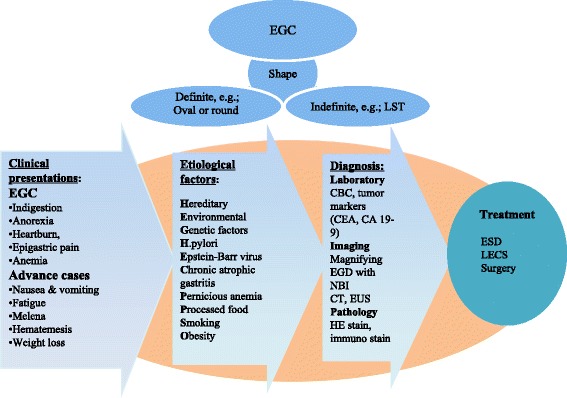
A summary of the clinical and aetiological factors and the management of early-gastric cancer lesions. (EGC; early gastric cancer, LST; laterally spreading tumour, EGD; Oesophagogastroduodenoscopy, CBC; complete blood count, NBI; narrow band imaging; CT; computed tomography, EUS; endoscopic ultrasound, HE; haemoxylin and eosin, CEA; Carcinoembryonic antigen, CA 19–9; Carbohydrate Antigen 19–9, ESD; endoscopic submucosal dissection, LECS; Laparoscopic and endoscopic cooperative surgery).
